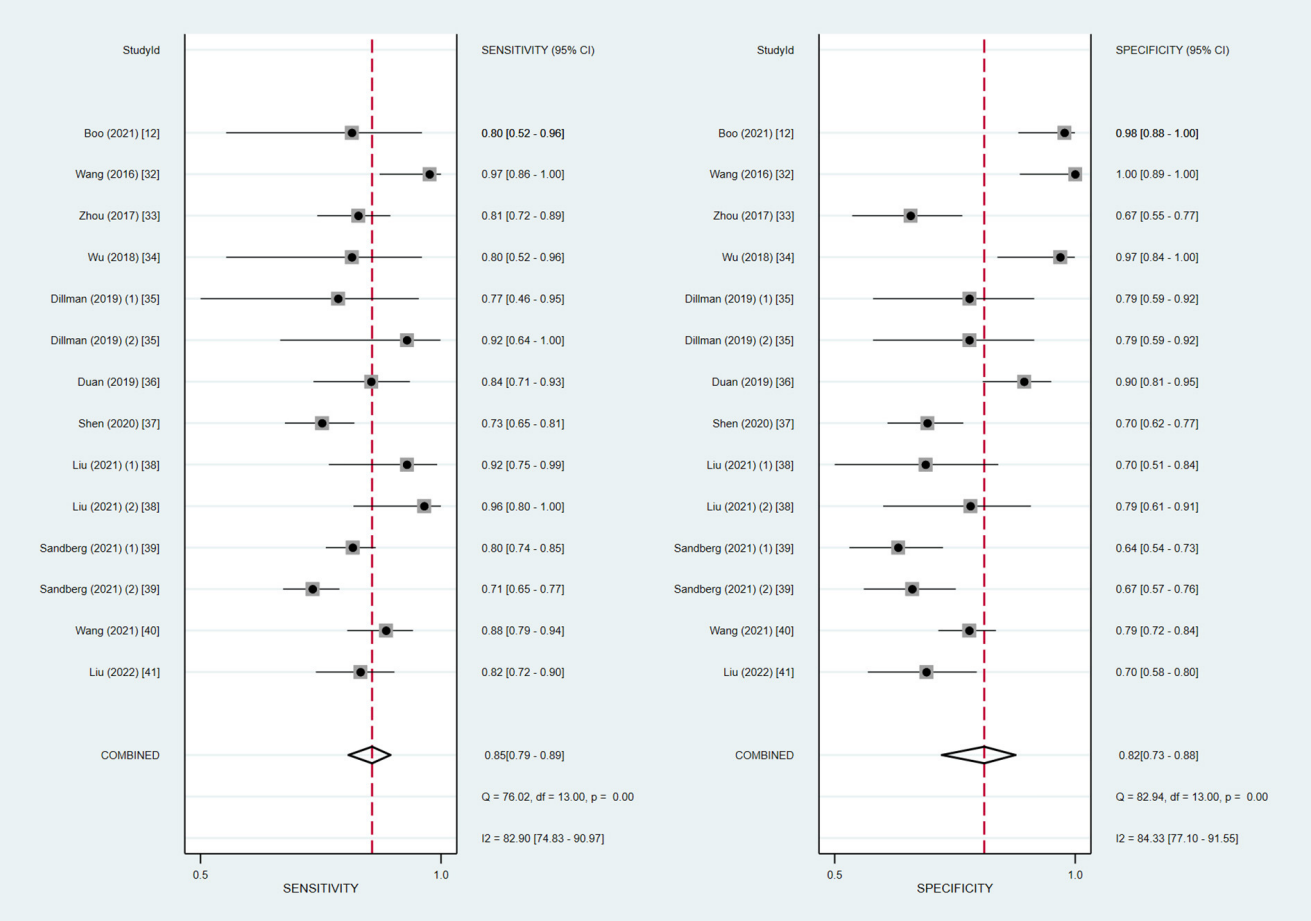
Coupled forest plots of the summary sensitivity and specificity of ultrasound elastography for the diagnosis of biliary atresia (BA).
